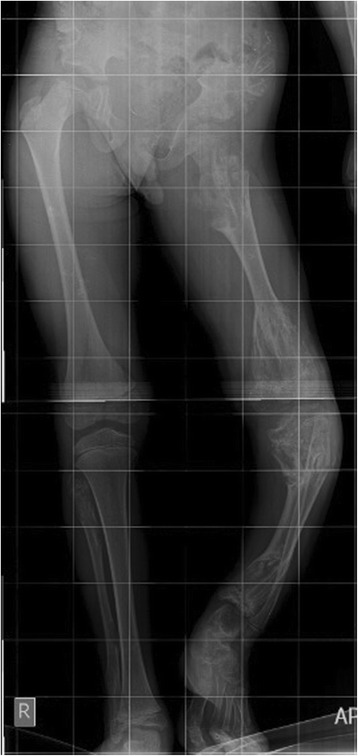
Anterior-posterior radiograph of pelvis and both lower limbs: enchondromas in the upper and lower ends of left femur, tibia, fibula as well as left iliac crest with left lower limb discrepancy and bowing deformity
